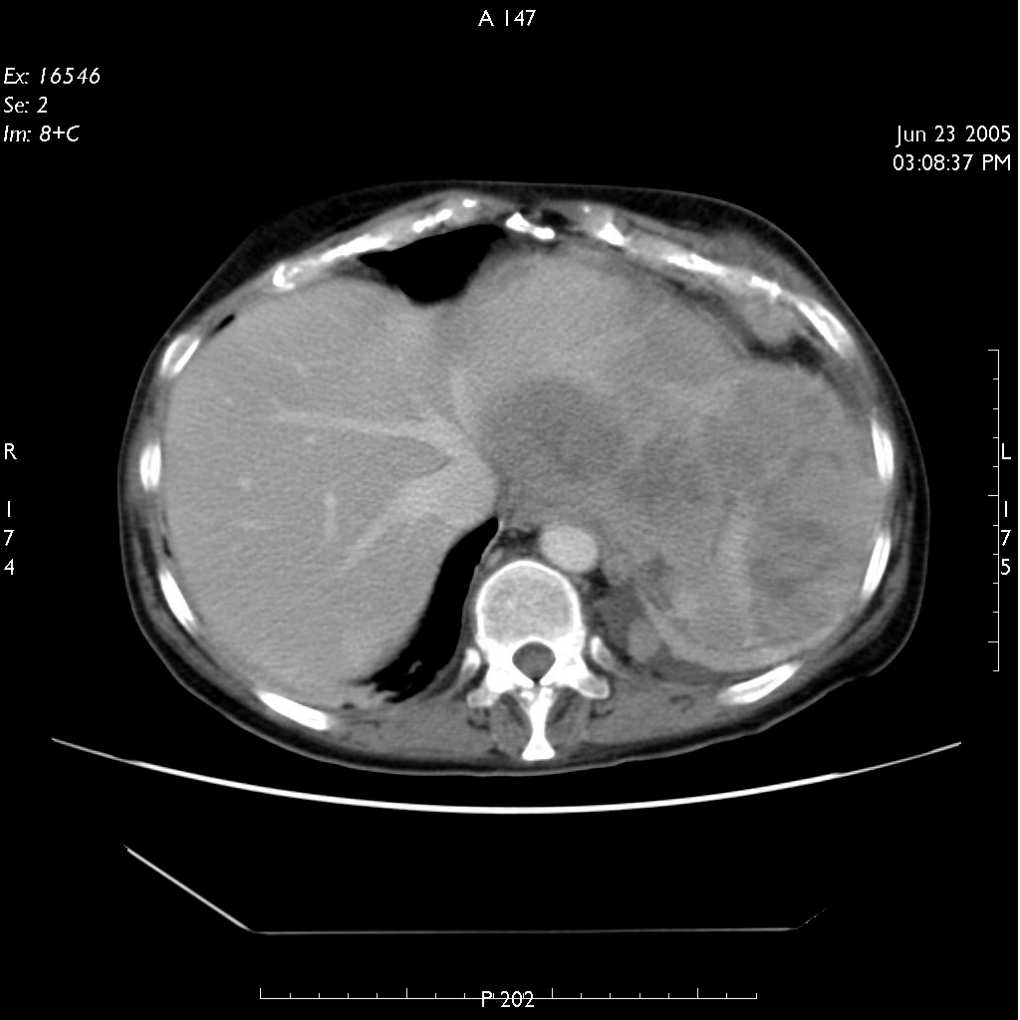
CT scan of abdomen showing local recurrence of the tumour.
